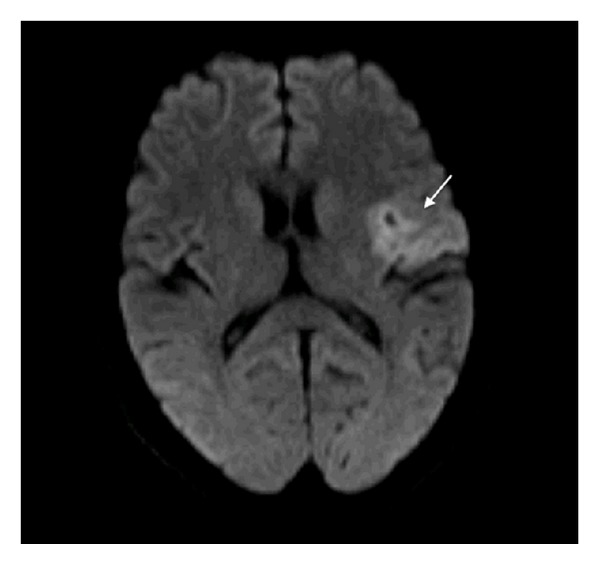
Axial diffusion MRI scan demonstrating high intensity lesion at the left frontal region consistent with acute infarction.
